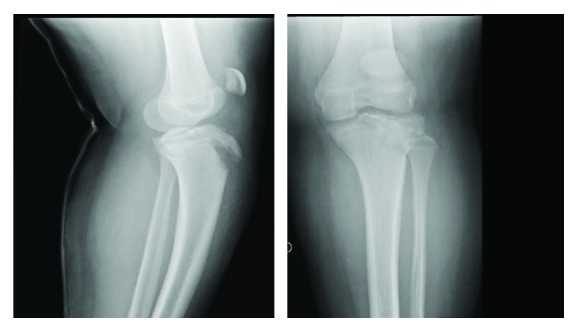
AP and lateral view X-ray of the left knee.
